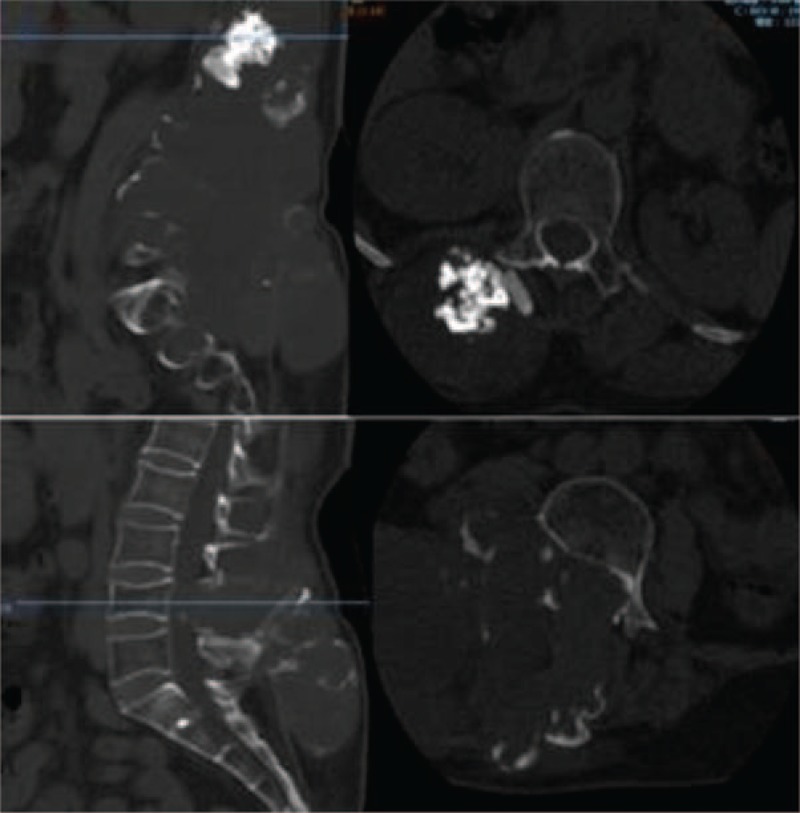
A CT scan revealed bone destruction in the adnexa of L1-S2 that was surrounded by lump-like abnormal density shadows. The CT intensity was approximately 9-53 HU. Multiple plaque-like calcifications ranging from T11 to S3 were also observed. CT = computed tomography.
